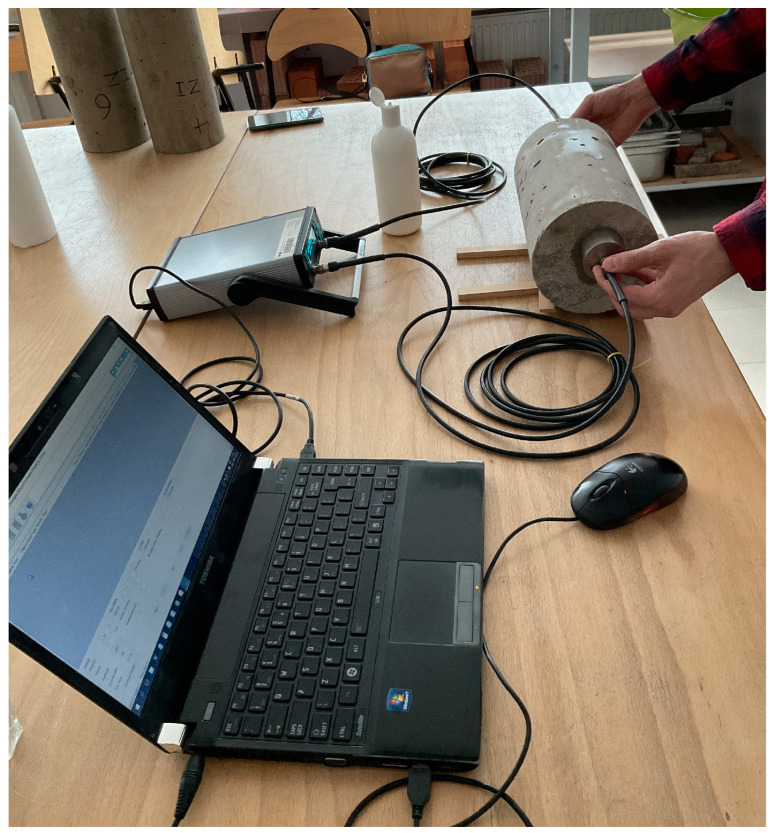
Testing equipment used for measurements of ultrasonic pulse velocity.
